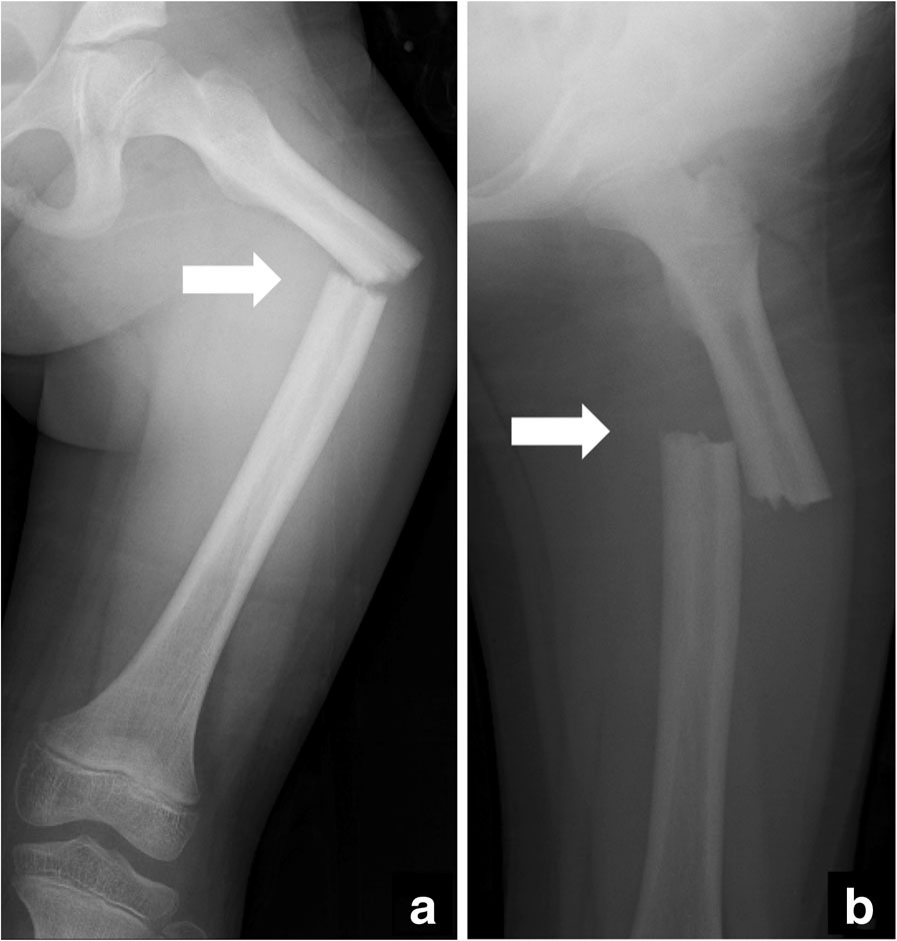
Radiographs of the left femur at the initial examination presenting a femoral shaft fracture (arrow). a the anteroposterior view, b the lateral view
